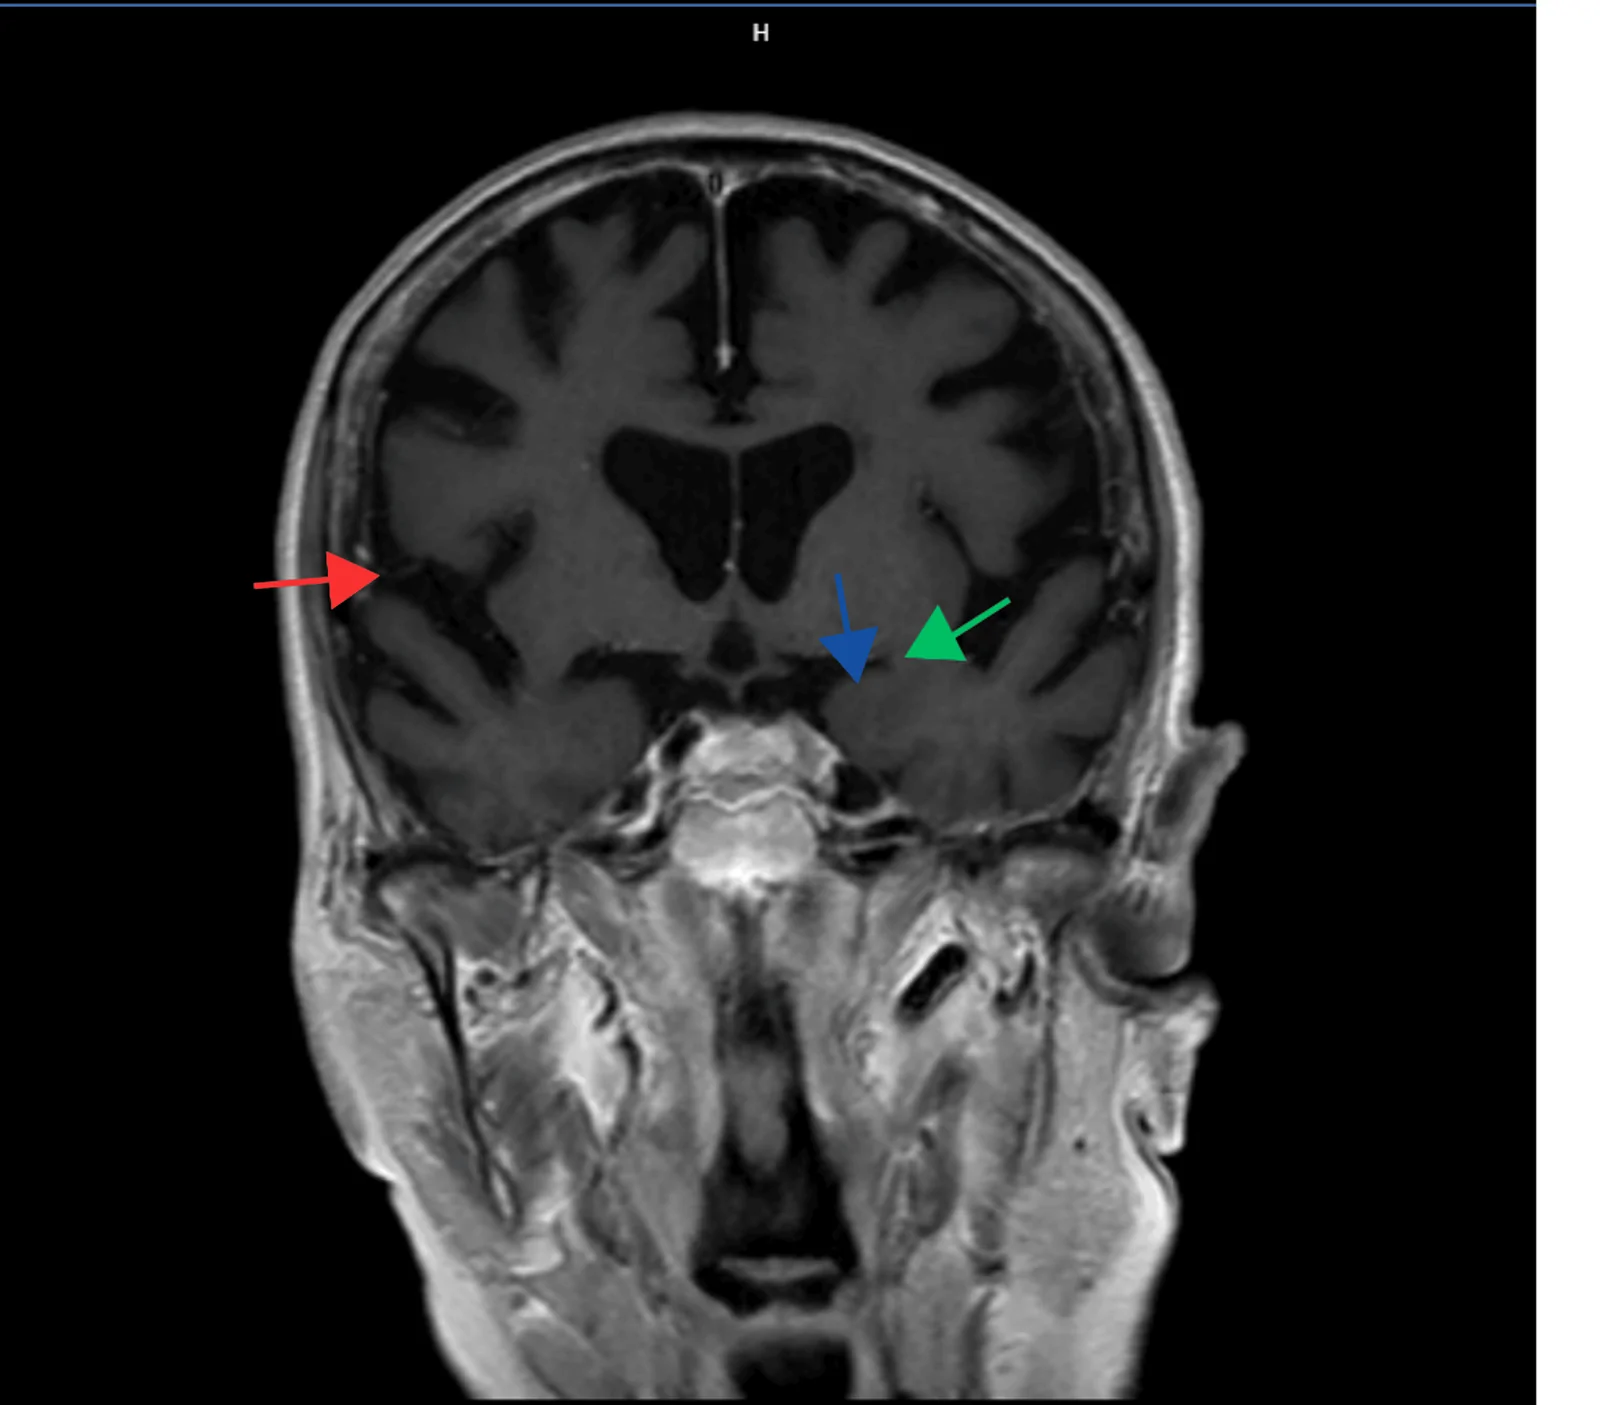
MRI brain: coronal T1 The MRI demonstrates generalized brain-volume loss (red arrow), with demonstration of the hippocampal region (blue arrow) and temporal horn (green arrow).
